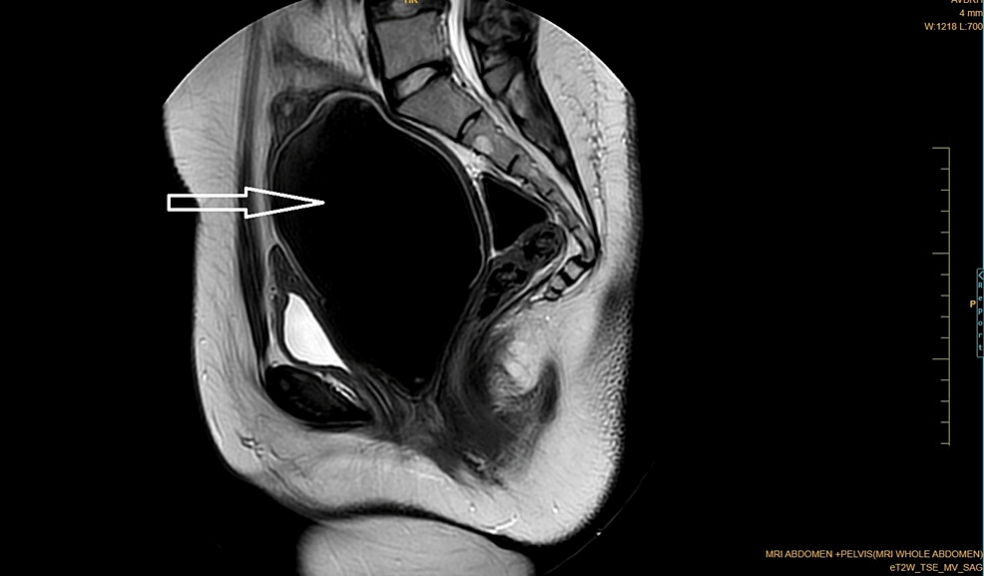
Sagittal T2WI showing large well-defined hypointense cystic collection inside the vagina. Note: Authors' original image T2WI: T2-weighted image
